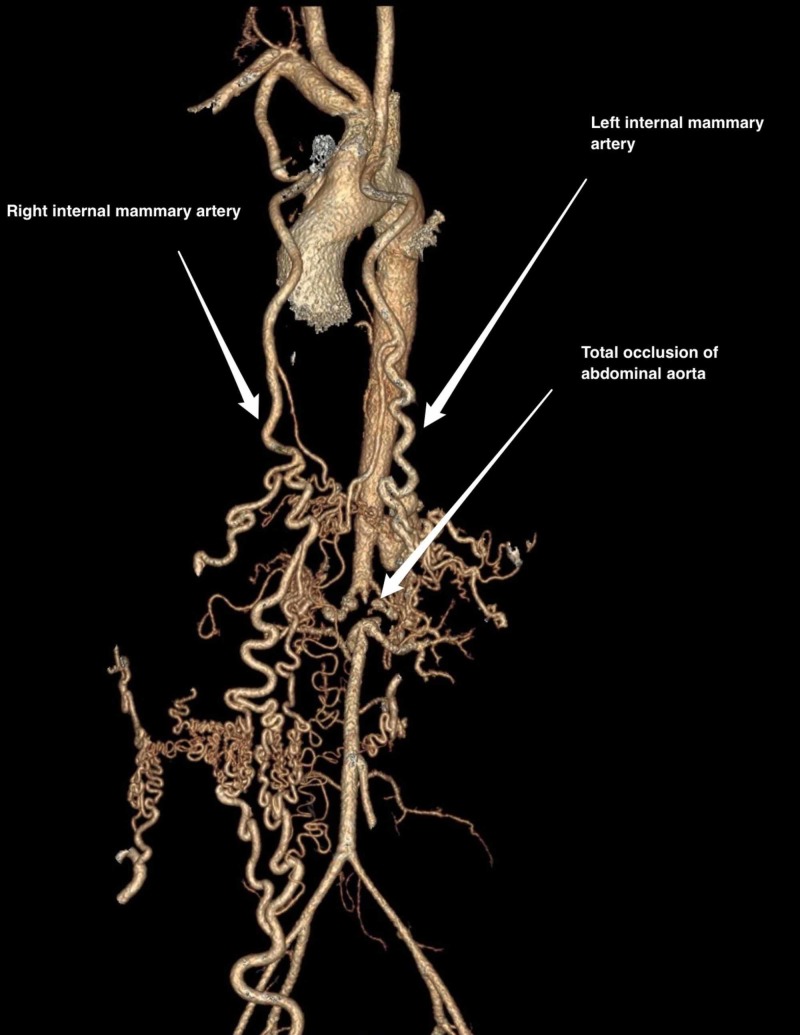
Computed tomography aortogram showing collateral vessels formed by the right and left internal mammary artery
